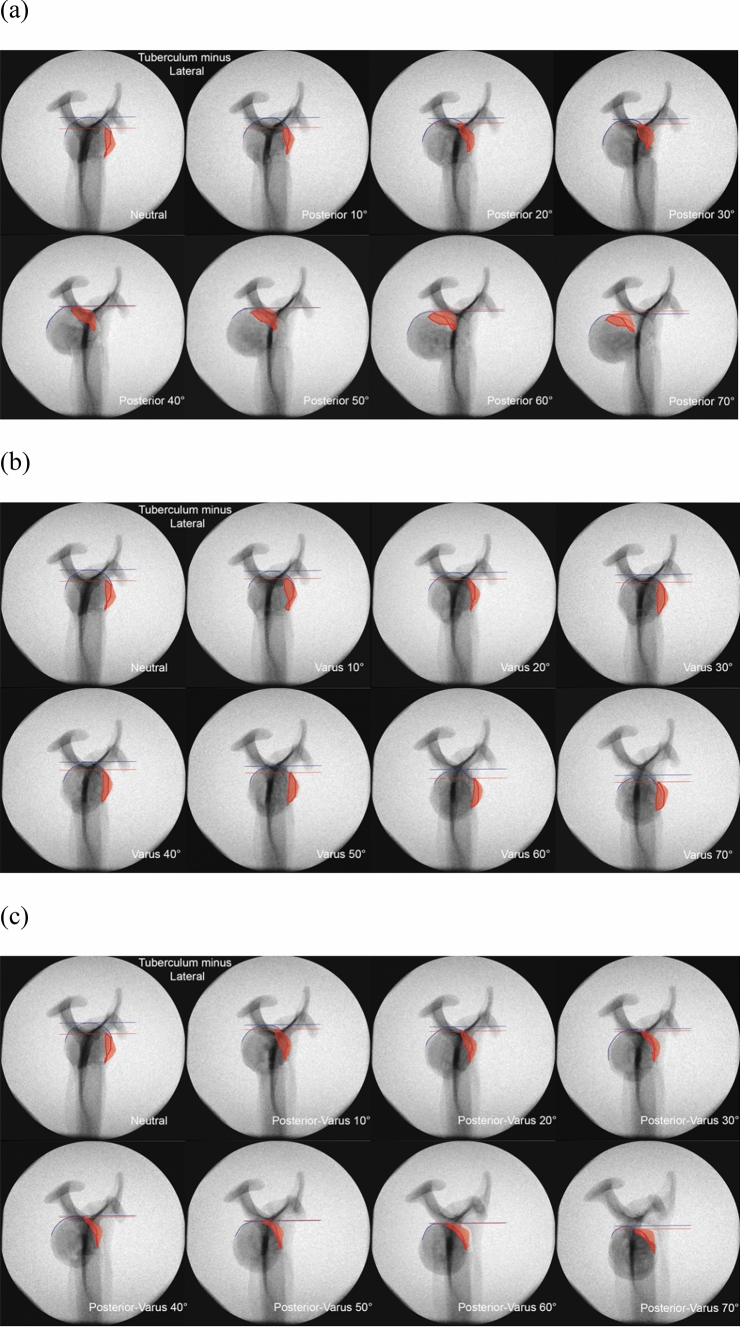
Scapular *Y*-view radiographic series of the lesser tuberosity (red) with increasing a posterior, b varus, and c combined posterior-varus angulation. Blue and red lines indicate the most proximal point on the head contour and tuberosity, respectively
